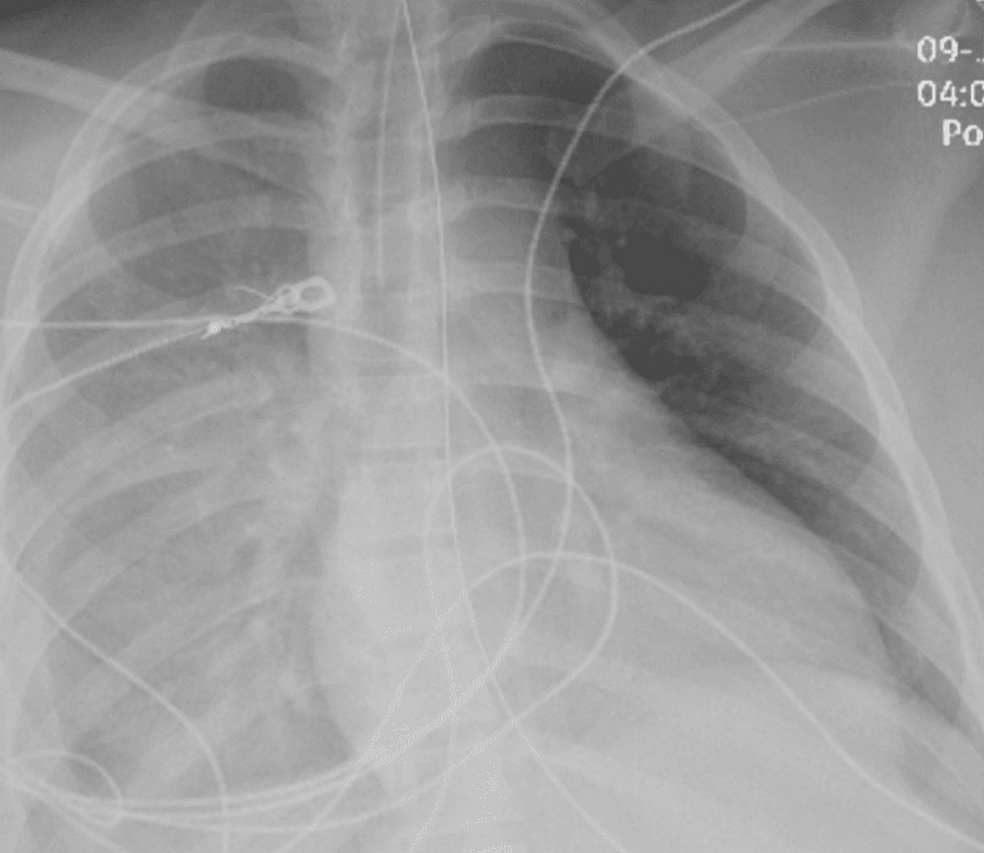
Chest X-ray on day three of admission showing near-complete opacification of the right hemithorax
